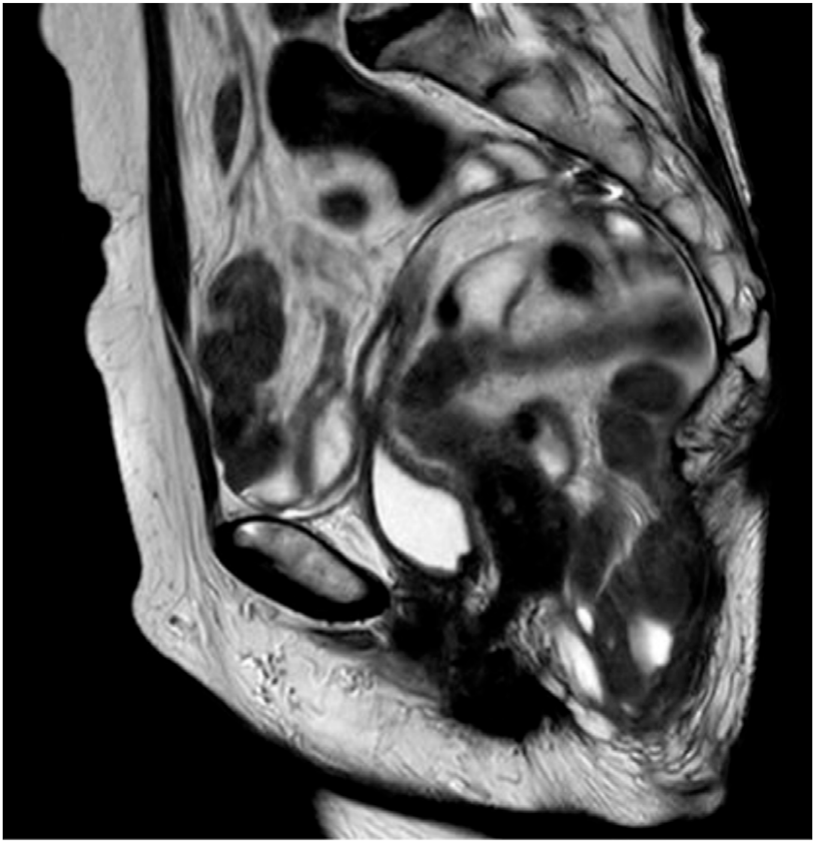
Post-operative MRI pelvis showing no perineal recurrence. Sagittal plane.
